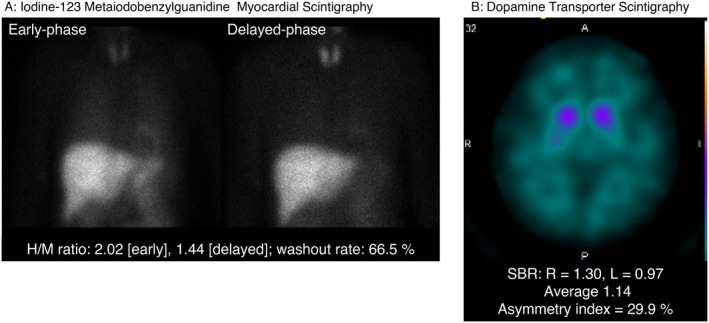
Nuclear medicine examinations in this case. (A) Iodine‐123 metaiodobenzylguanidine (^123^I‐MIBG) myocardial scintigraphy (left: Early phase; right: Delayed phase) showing reduced cardiac uptake on delayed imaging (heart‐to‐mediastinum [H/M] ratio: 2.02 [early], 1.44 [delayed]; washout rate: 66.5%). For reference, H/M ratios > 2.20 (early and delayed) and a washout rate < 34% are considered to be within the normal range. (B) Dopamine transporter scintigraphy (^123^I‐FP‐CIT SPECT; DaTSCAN) showing reduced bilateral striatal tracer uptake. (specific binding ratio [SBR]: Right, 1.30; left, 0.97; mean, 1.14; asymmetry index, 29.9%).
